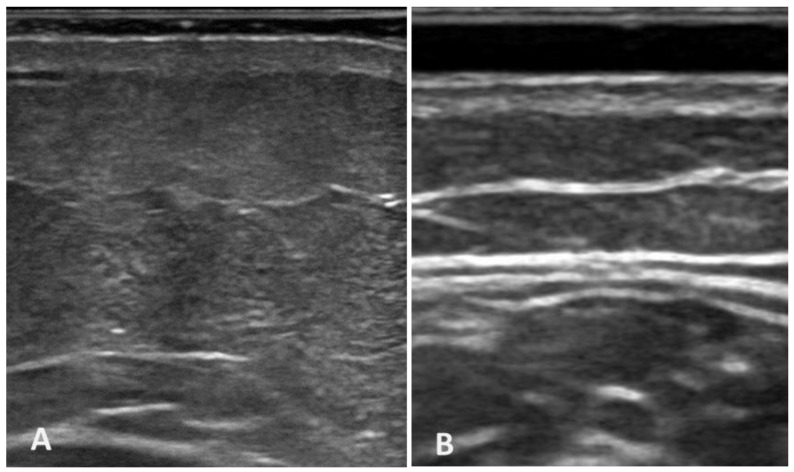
Ultrasound imaging of superficial and deep fasciae in chronic lymphedema patients and healthy volunteers. (A) Ultrasound image of chronic lymphedema, displaying thin superficial and deep fasciae. This reduced thickness is characteristic of chronic lymphatic stasis, which is likely due to tissue remodeling associated with prolonged lymphedema. (B) Ultrasound image of healthy volunteers, showing good visibility of superficial and deep fasciae, highlighting the structural integrity of the fasciae and subcutaneous tissue.
